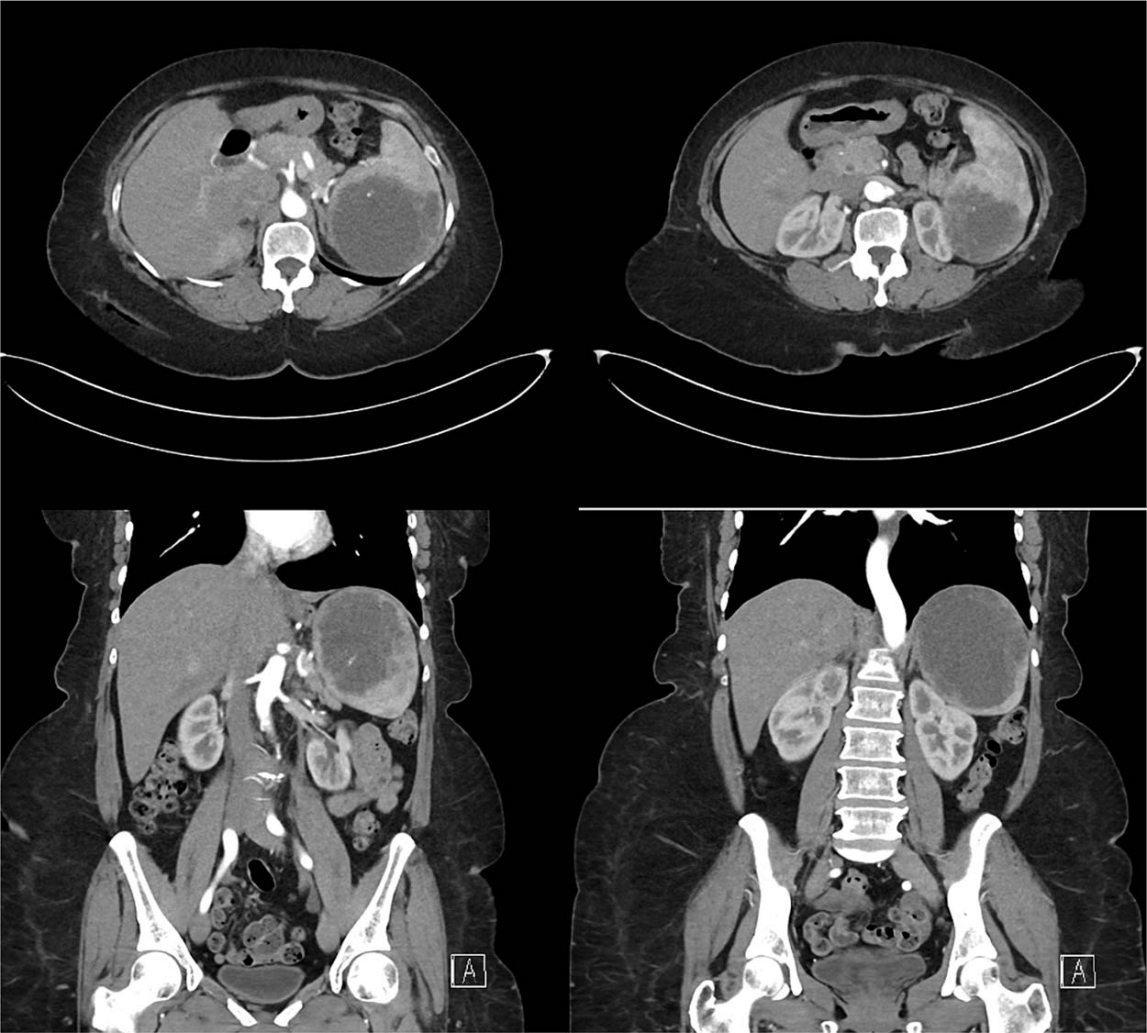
Abdominal and pelvic CT scan revealed a large, non-enhancing hypodense cystic lesion in the spleen with internal septations, calcifications, and multiple smaller daughter cysts arranged peripherally. Prime differential was type II hydatid cyst.
